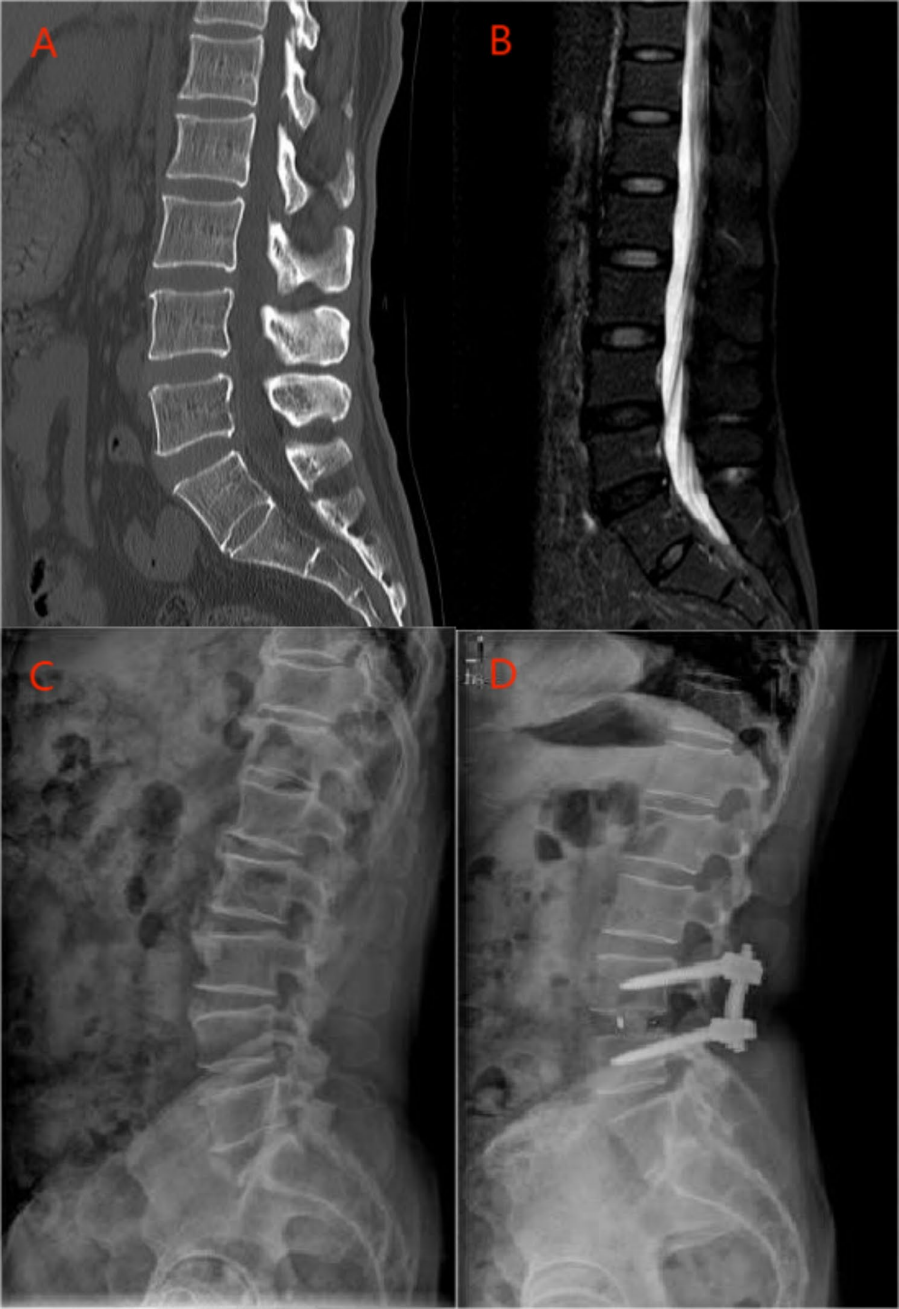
Preoperative sagittal computed tomographic scan (A), sagittal T2-weighted magnetic resonance image (B), sagittal lateral view (C), and sagittal lateral view at the final follow-up (D) of a 51-year-old female patient with L4/5 degenerative lumbar spinal stenosis treated with lumbar interbody fusion
